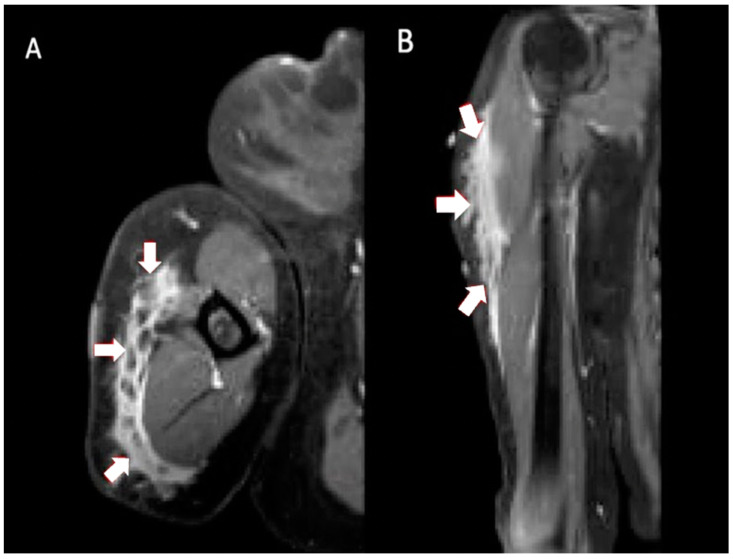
Multiplanar MRI of right shoulder taken 8 months after vaccine administration in right shoulder. Axial T2 (A) and coronal T2 (B) images show ill-defined fluid signal surrounding interspersed areas of fat. The involved area extended from surgical neck of the humerus to distal diaphysis, measuring approximately 14.9 × 8.2 × 1.8 cm (arrows).
